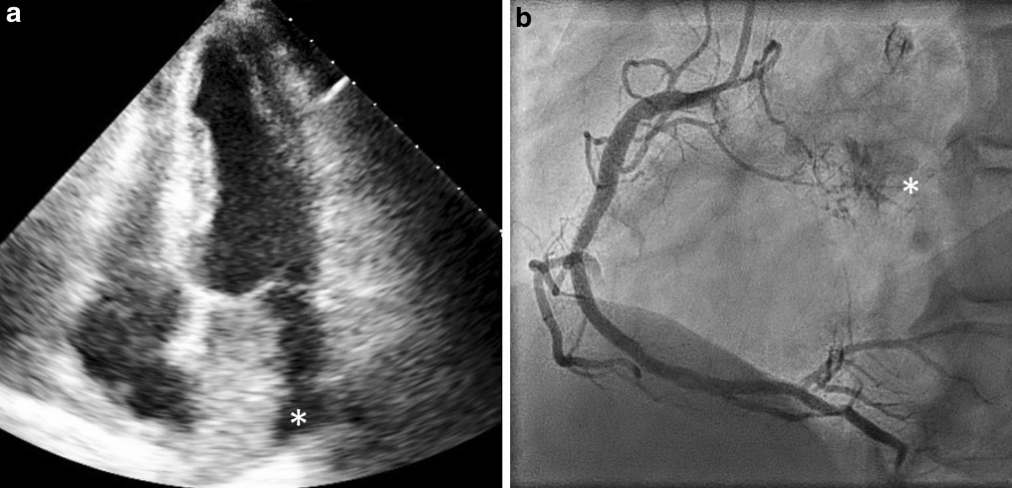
a Echocardiogram showing cardiac mass in left atrium. b Coronary angiogram showing supply to an extra-cardiac mass from the right coronary artery
